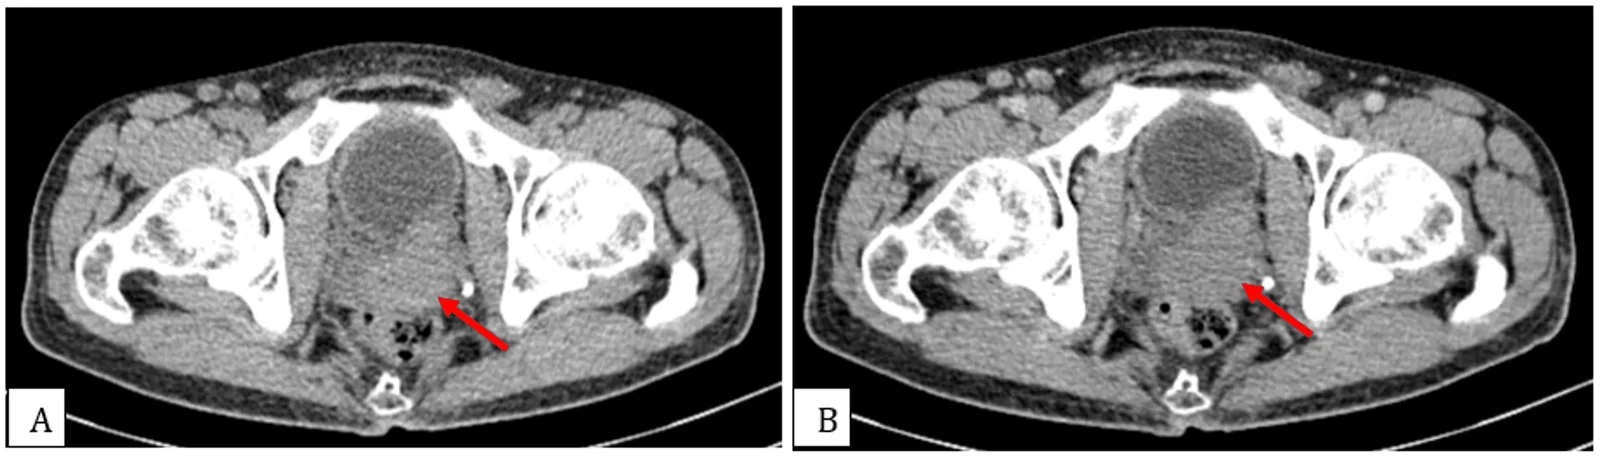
Axial computed tomography (CT) images of the pelvis. (A) Unenhanced image demonstrating a well-defined, homogeneous hyperattenuating cystic lesion (attenuation 52 HU) centered on the left seminal vesicle (red arrow). (B) Contrast-enhanced image showing unchanged attenuation (52 HU; ΔHU = 0) with no appreciable enhancement of the lesion (red arrow), confirming its non-solid nature. HU: Hounsfield units
